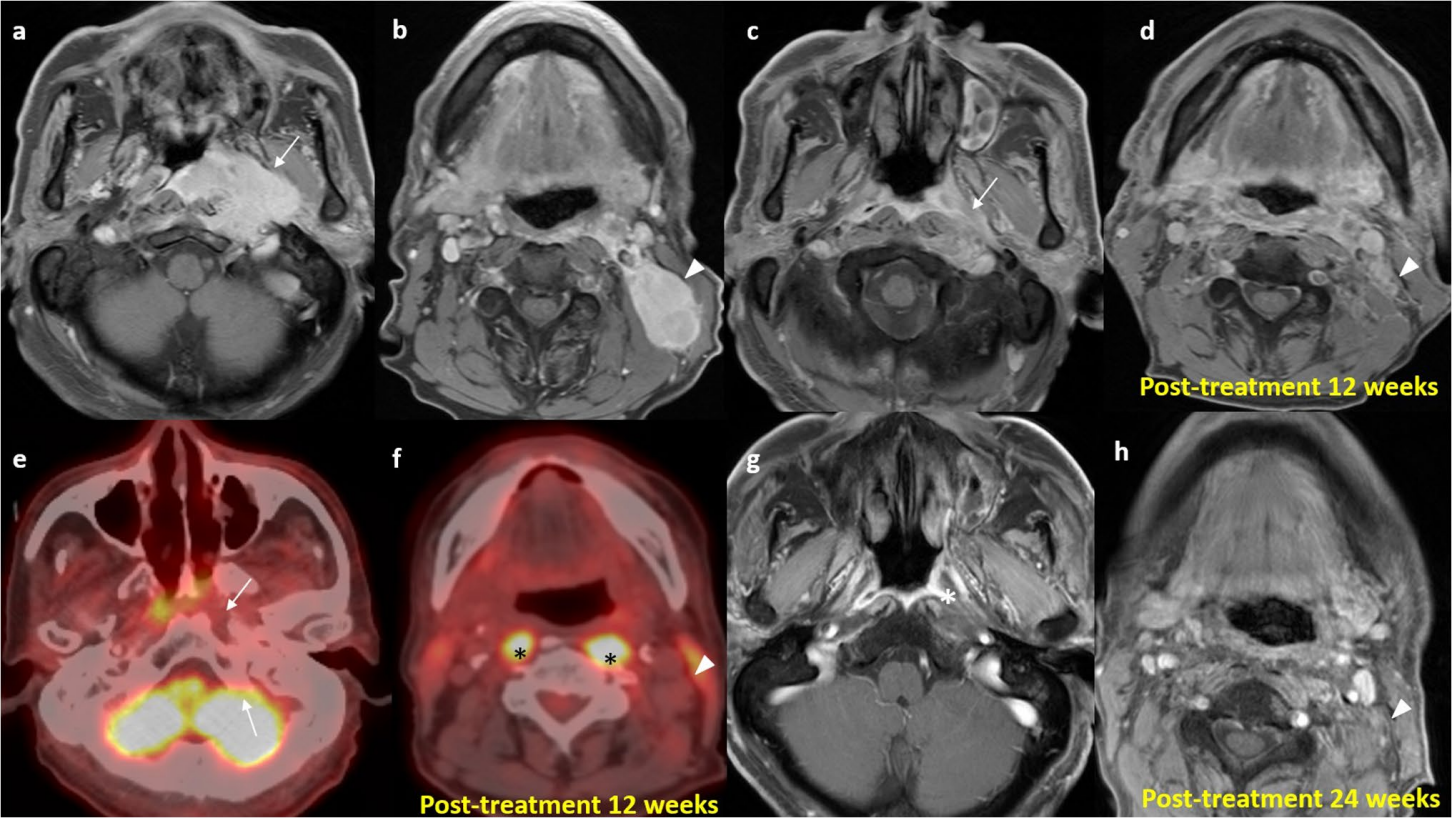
Delayed tumor regression and time lag between anatomical and metabolic regression in a 69-year-old man with locally extensive NPC (clinical stage T4N3M0) completed chemo-irradiation in 2023. Axial T1 post-contrast images with fat-suppression at pre-treatment stage show a large and infiltrative enhancing left nasopharyngeal tumor (arrow) and left level II lymphadenopathy (arrowhead) (a) & (b). There was evidence of pathological complete remission in the nasopharyngeal tumor at 10 weeks after completion of treatment. Axial T1 post-contrast images with fat suppression at 12 weeks show interval shrinkage in size of the known tumor with residual enhancing soft tissue in the left retropharyngeal and prevertebral space (arrow). The left level II node also shows interval shrinkage with residual heterogeneously enhancing soft tissue (arrowhead) (c) and (d). These were equivocal for residual tumors, which might warrant further palliative treatment. Axial fusion FDG PET/CT images at 12 weeks show the left nasopharyngeal lesion (arrow) as well as the left level II lesion (arrowhead) to be non-FDG avid, suggestive of metabolic resolution, despite the small residual soft tissue density. Apparent muscle activity along the bilateral longus colli muscles (asterisks) is a known diagnostic pitfall mimicking disease recurrence (e) and (f). FDG PET/ CT can be a helpful tool in this occasion due to its high negative predictive value. The patient subsequently underwent clinical observation instead of unnecessary biopsy or oncological therapy. Axial T1 post-contrast images at 24 weeks show almost complete resolution of the left nasopharyngeal lesion (asterisk) and the left level II node (arrowhead) (g) and (h)
